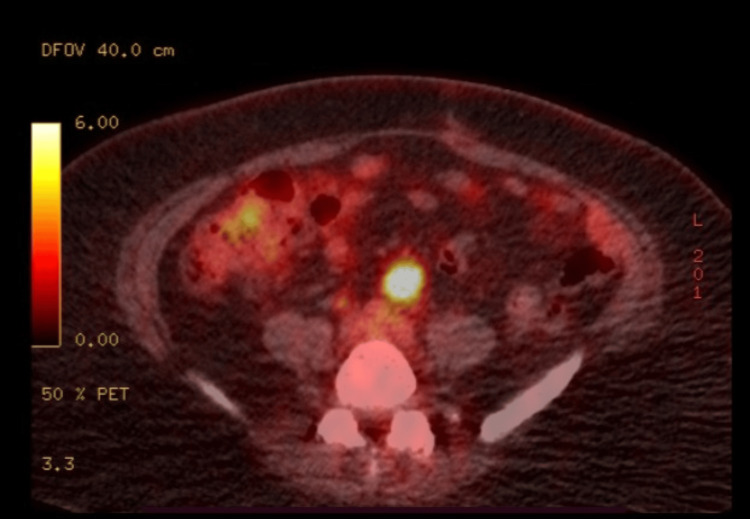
Hypermetabolic lymph node anterior to the aortic bifurcation as observed on the skull base to mid-thigh PET/CT scanning at the six-month follow-up PET/CT, positron emission tomography/computed tomography
